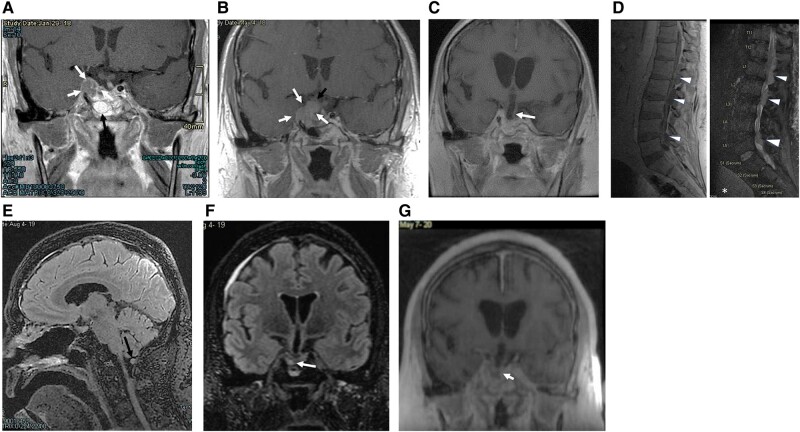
(A) Post gadolinium T1 coronal MRI following first resection in 2017. Recurrent tumor highlighted with white arrows. Enhancing mucosa in the sphenoid sinus (black arrow). (B) Post gadolinium T1 coronal MRI prior to second surgery in 2018. Enlargement of tumor (white arrows) encroaching on chiasm and right optic nerve (black arrow). (C) Post gadolinium T1 coronal MRI after second resection in 2018. Significant tumor debulking; interval lateral and third ventricular enlargement with enlarged third ventricle extending into sella (white arrow) (D) T1 sagittal (3A) and FSEIR sagittal (3B) MRI at metastases of prolactin-secreting pituitary carcinoma showing multiple epidural masses in lumbar spine at L1, L2-3, and L4-5 with dural compression. Note enlarged bladder due to cauda equina compression (asterisk). (E and F) At diagnosis of pituitary carcinoma in August 2019. FLAIR sagittal and coronal. No significant change in right-sided intrasellar tumor (white arrow). More bulk of left-sided pituitary tissue. Metastatic nodule inferior to cerebellar tonsil (black arrow). (G) Post gadolinium T1 coronal at prolactin nadir in May 2020. Tumor residual remains small (white arrow). Abbreviation: MRI, magnetic resonance imaging.
